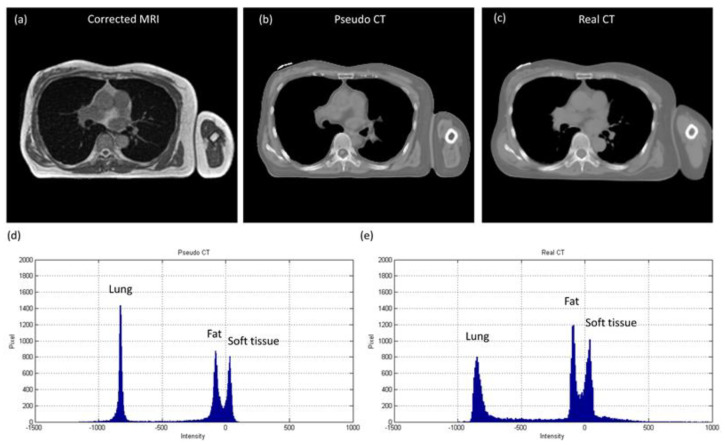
(a) Corrected MRI from 0.35 T scanner (WL/WW = 100/200), (b) pseudo-CT (WL/WW = 0/1000), (c) real CT (WL/WW = 0/1000), (d) histogram of pseudo-CT, and (e) histogram of real CT for chest imaging used to generate CNN validation dataset (${\mathrm{IMG}}_{\mathrm{validation}}^{\mathrm{chest}}$).
